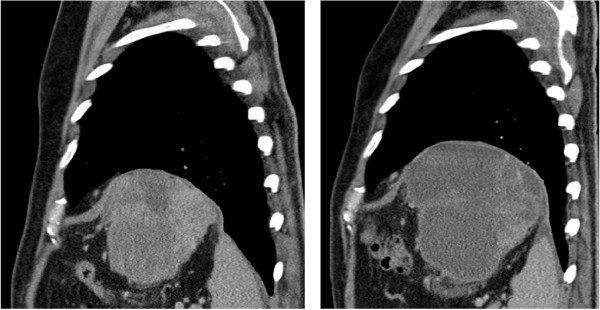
Sagittal CT image of left upper quadrant mass. Although the mass has increased in size, the drop in Hounsfield units from 39 to 16 suggests partial response by Choi criteria.
